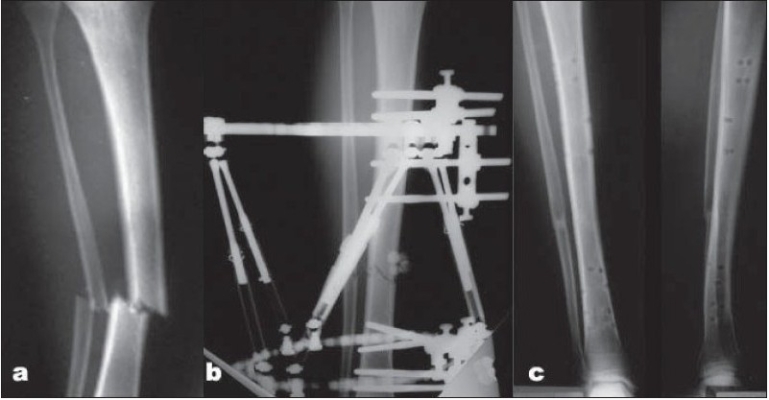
(a) Radiograph showing tibial shaft fracture in a 20 years old male patient (case 5). (b) Anteroposterior radiograph immediately after application of TSF with reduction of the fracture (c) Anteroposterior and lateral radiographs 6 months after removal of fixator showing radiological union.
